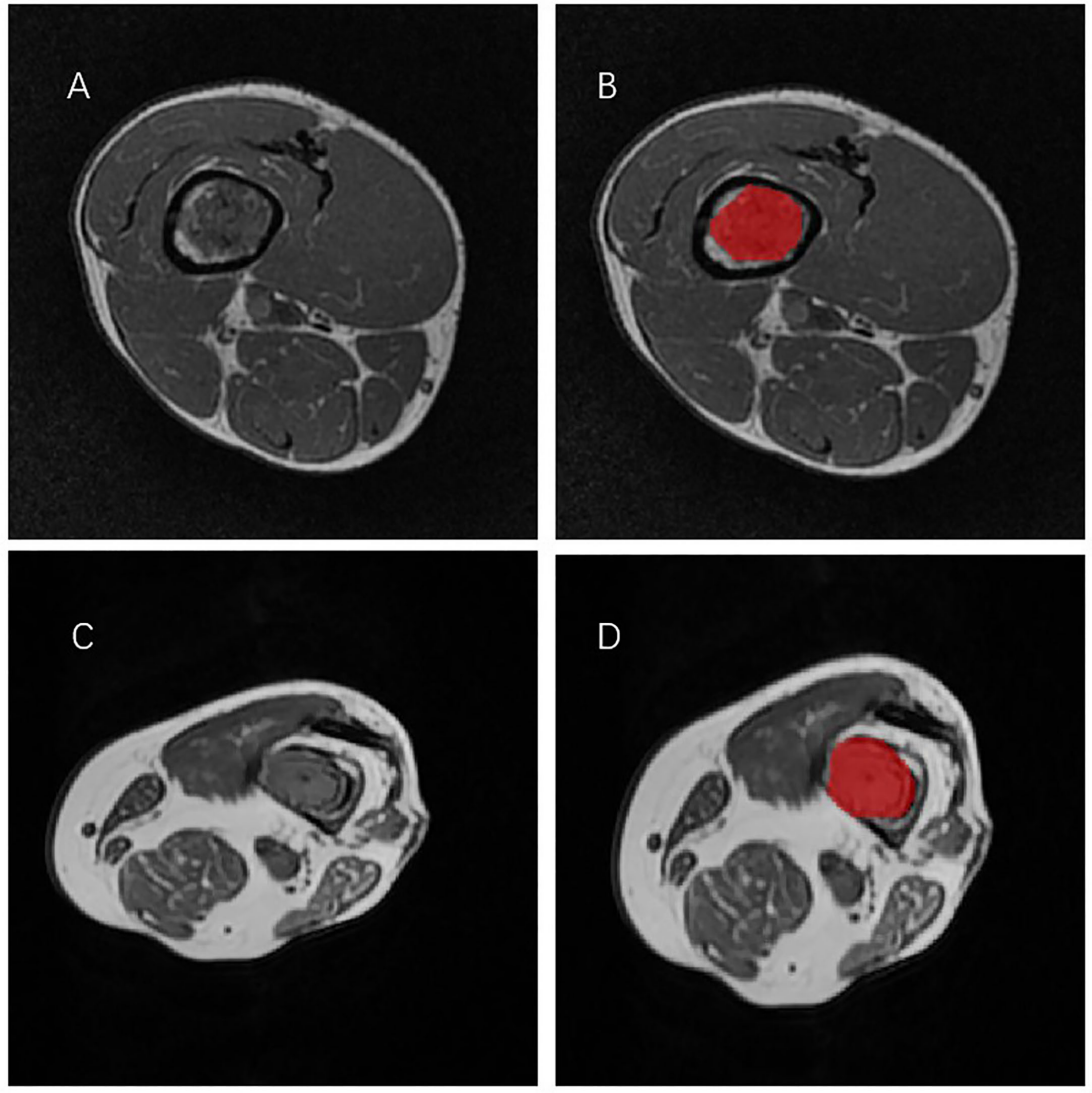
Two representative T1WI MRI segmentation on ITK-SNAP. (A, B) A patient with low-grade chondrosarcoma of the tibia. (C, D) A patient with high-grade chondrosarcoma of the tibia.
